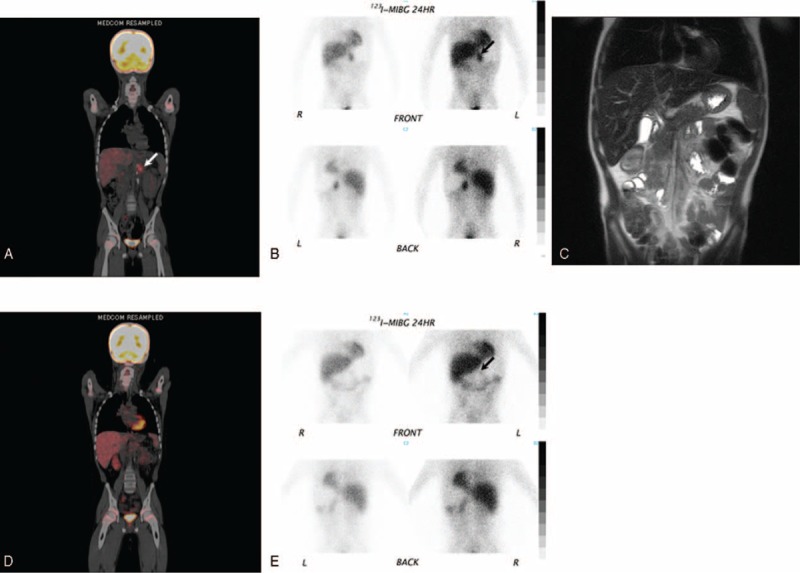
Images of an abdominal mass in an 8-year-old boy. (A) Coronal fused ^18^F-FDG-PET and CT before 13-*cis*-RA treatment. ^18^F-FDG accumulation is indicated by the arrow. (B) Planar ^123^I-MIBG scintigraphy before 13-*cis*-RA treatment. Tracer accumulation is indicated by the arrow. (C) No residual tumor is observed on T2-weighted coronal MRI acquired before 13-*cis*-RA treatment. (D) No ^18^F-FDG accumulation is observed 18 months after the initiation of 13-*cis*-RA treatment. (E) Faint ^123^I-MIBG uptake (arrow) is seen 18 months after the initiation of 13-*cis*-RA treatment. CT = computed tomography, 13-*cis*-RA = 13-*cis*-retinoic acid, ^18^F-FDG-PET = ^18^F-fluorodeoxyglucose positron emission tomography, ^123^I-MIBG = iodine-123 metaiodobenzylguanidine, MRI = magnetic resonance imaging.
